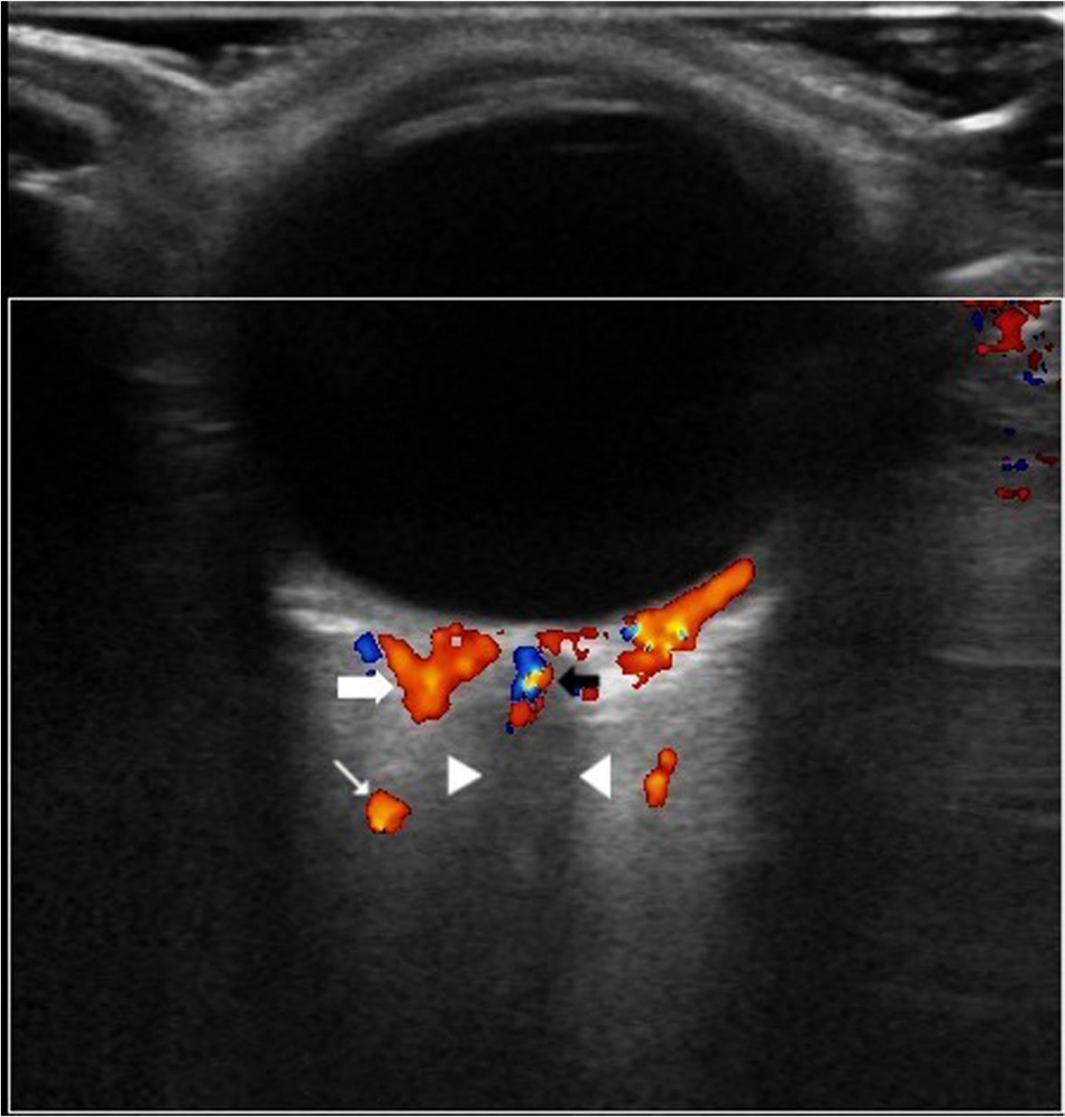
Axial doppler ultrasound section of left eye and orbit. Ophthalmic artery (white thin arrow), short posterior ciliary artery (white thick arrow), central retinal artery-vein (black arrow) and optic nerve shadow (arrowheads) can be seen posterior to the globe
